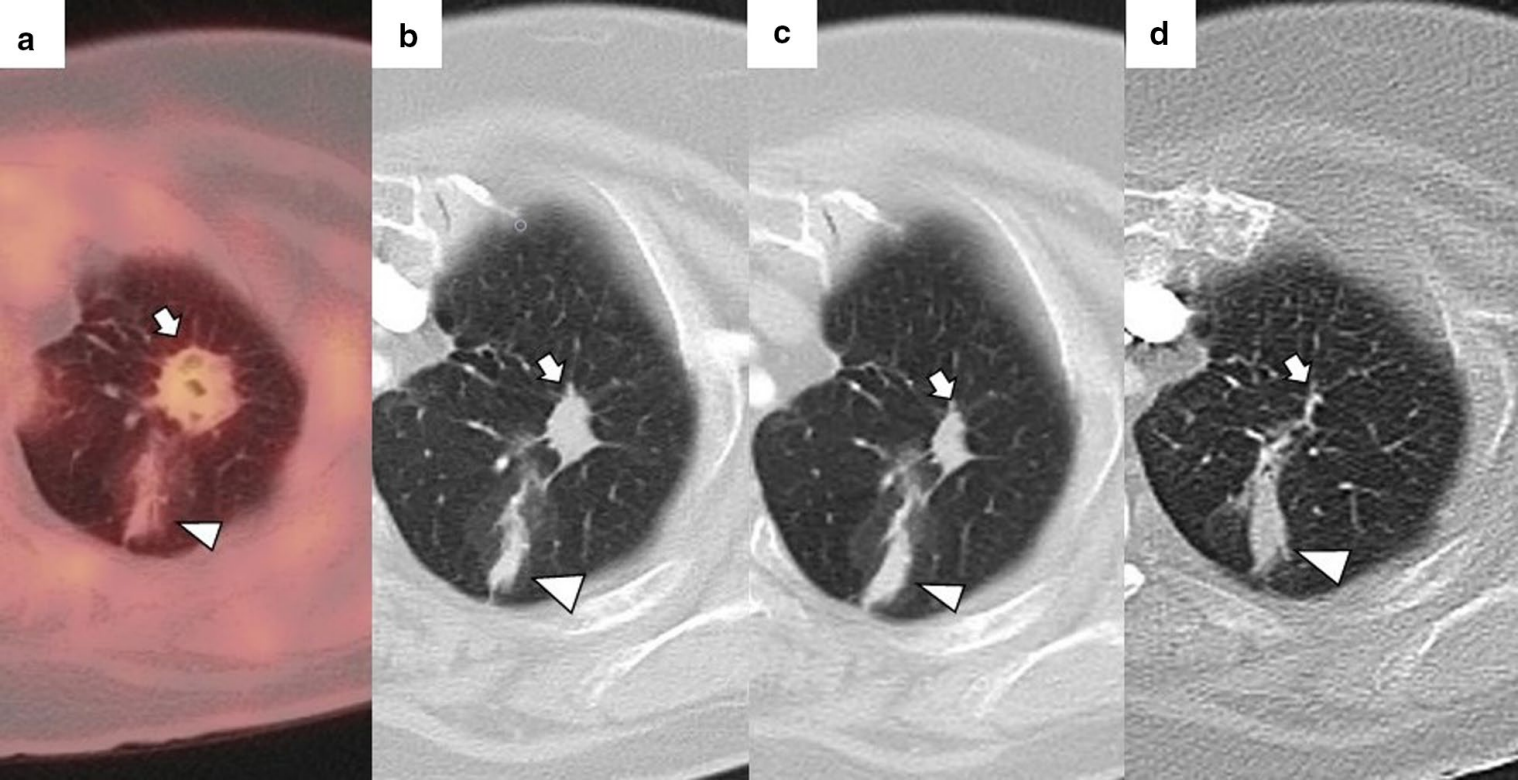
FDG uptake in a PET/CT one month after image-guided percutaneous lung ablation. a PET-CT acquired after one month of percutaneous lung MWA, showing a growth of the ablative area compared to the pre-treatment CT (Fig. 7-A). It also presented a subtle uptake of FDG within the treated area (arrow). Given the proximity of the treatment, this is probably due to an inflammatory reaction. We also observe a residual scar due to a past probe-path-related lung hemorrhage reported (triangle). b–d We observed a progressive reduction in the size of the ablative area after 3 (b), 6 (c), and 12 months (d) of treatment, finally observing a residual scar (arrows)
